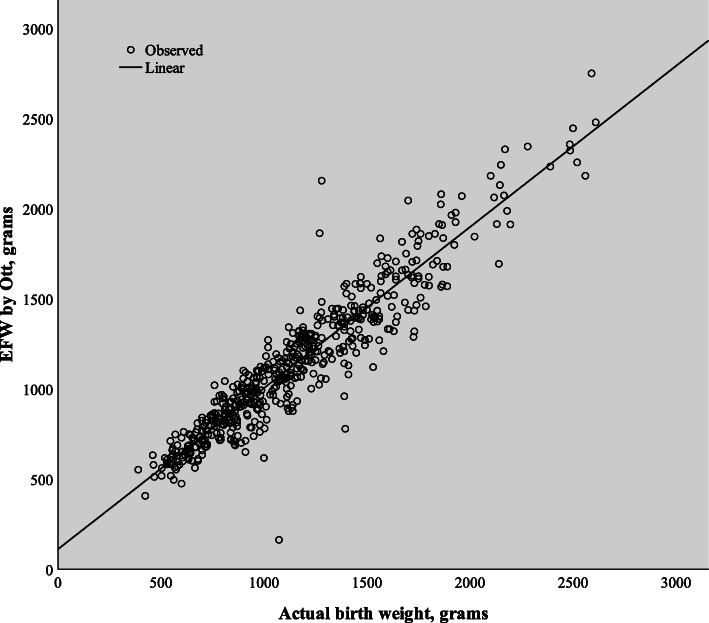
Correlation between sonographic estimated fetal weight and actual birth weight for the Ott formula (*r* = 0.884)
